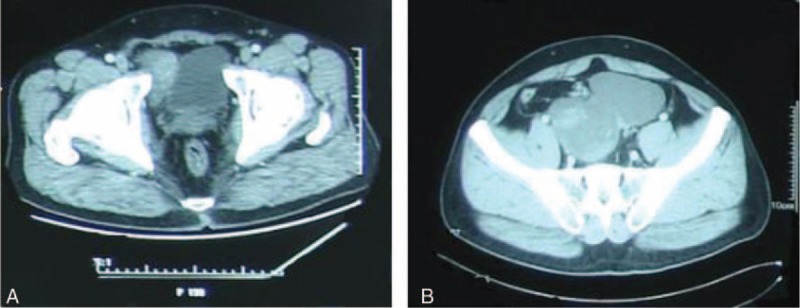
CT findings of the tumor in 2007. (A) CT showed a classical triangle soft tissue density shadow in the pelvic cavity; maximum cross-section was ∼5.5 × 2.5 cm. Lesions border was clear; its signal was well-distributed. Double side basin wall and inguinal region did not see obvious swelling lymph nodes; CT findings of the tumor at the second recurrence in 2009. (B) CT showed an irregular soft tissue mass shadow in the pelvic cavity. Lesions border was not clear. Local and right side of the bladder wall had not clear boundaries. CT = computed tomography.
